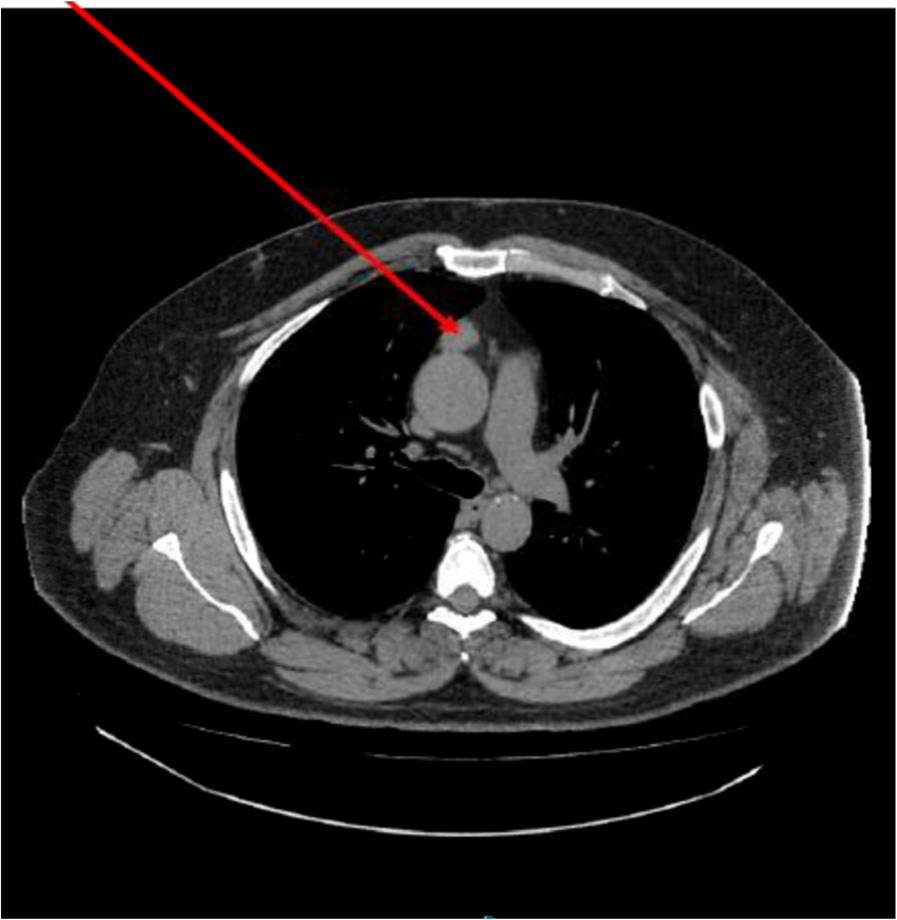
Arrow indicating anterior mediastinal mass on CT imaging
